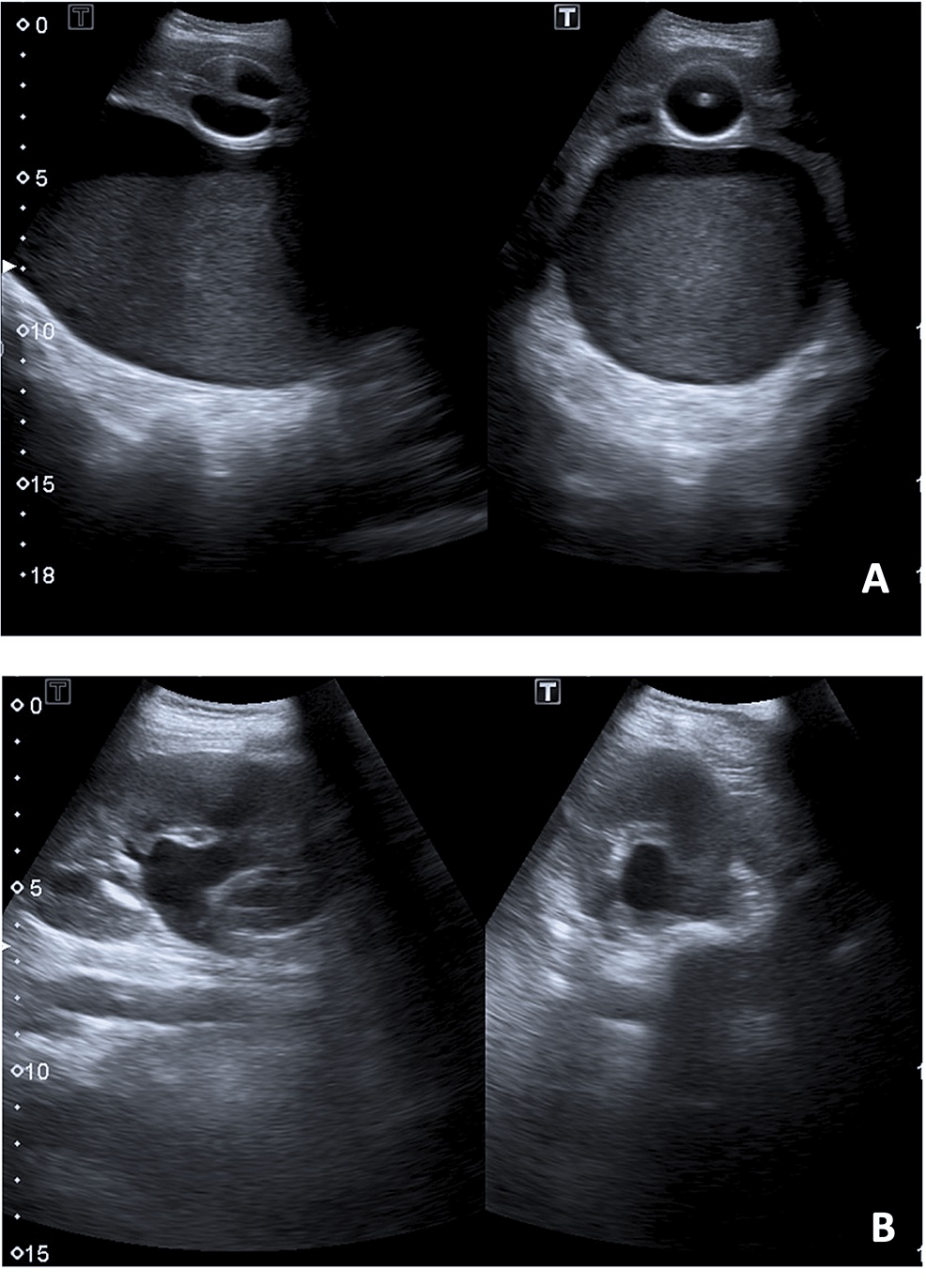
Abdominal and pelvic ultrasound A: Vaginal distension with hemorrhagic content (note: examination performed after bladder catheterization); B: Hydronephrosis
